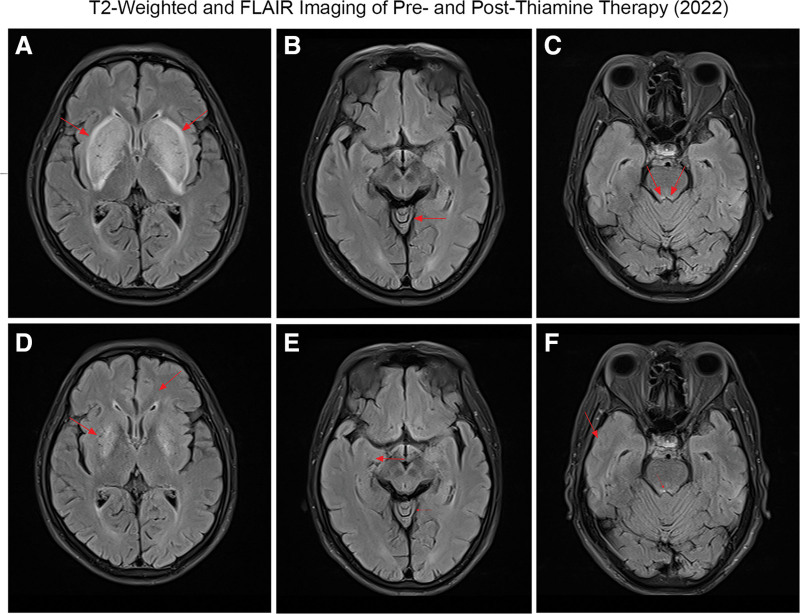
On the 1st hospitalization, red arrows indicated hyperintense signals in bilateral basal ganglia lesions, representing the “lentiform fork sign” (A), the cerebellar vermis (B), and the dorsal pons (C) on T2-weighted and fluid-attenuated inversion recovery (FLAIR) images. After thiamin therapy, hyperintense signals were decreased in bilateral basal ganglia lesions (D), the cerebellar vermis (E), and the dorsal pons (F) on T2-weighted and FLAIR images.
